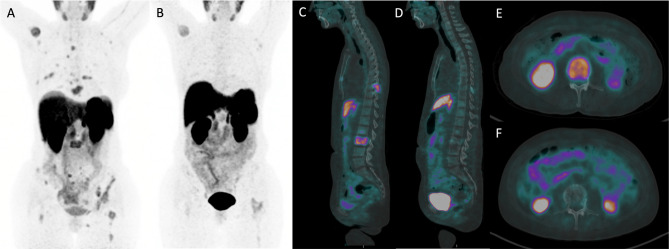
^68^Ga-DOTATATE PET/CT images displaying tracer uptake changes in a patient treated with selpercatinib. A, C, E = pre-TKI ^68^Ga-DOTATATE PET/CT. B, D, F = post-TKI ^68^Ga-DOTATATE PET/CT
